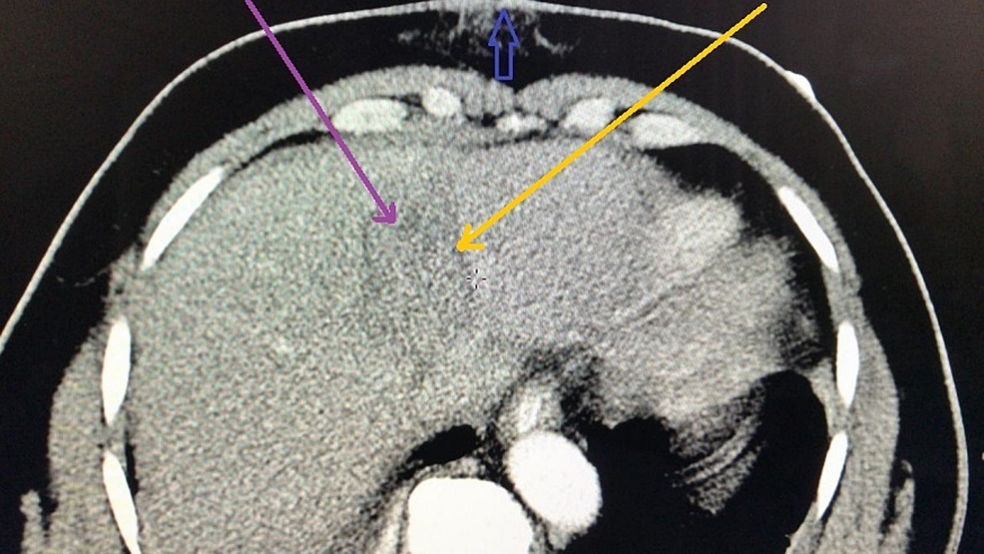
CT scan of abdomen and pelvis with intravenous contrast showing a grade Ⅲ liver injury through segment Ⅷ (blue arrow showing penetration of the skin and subcutaneous tissue of anterior abdominal wall; the purple and yellow arrows showing the non-enhancing low attenuation area with associated intra-parenchymal haematoma)
